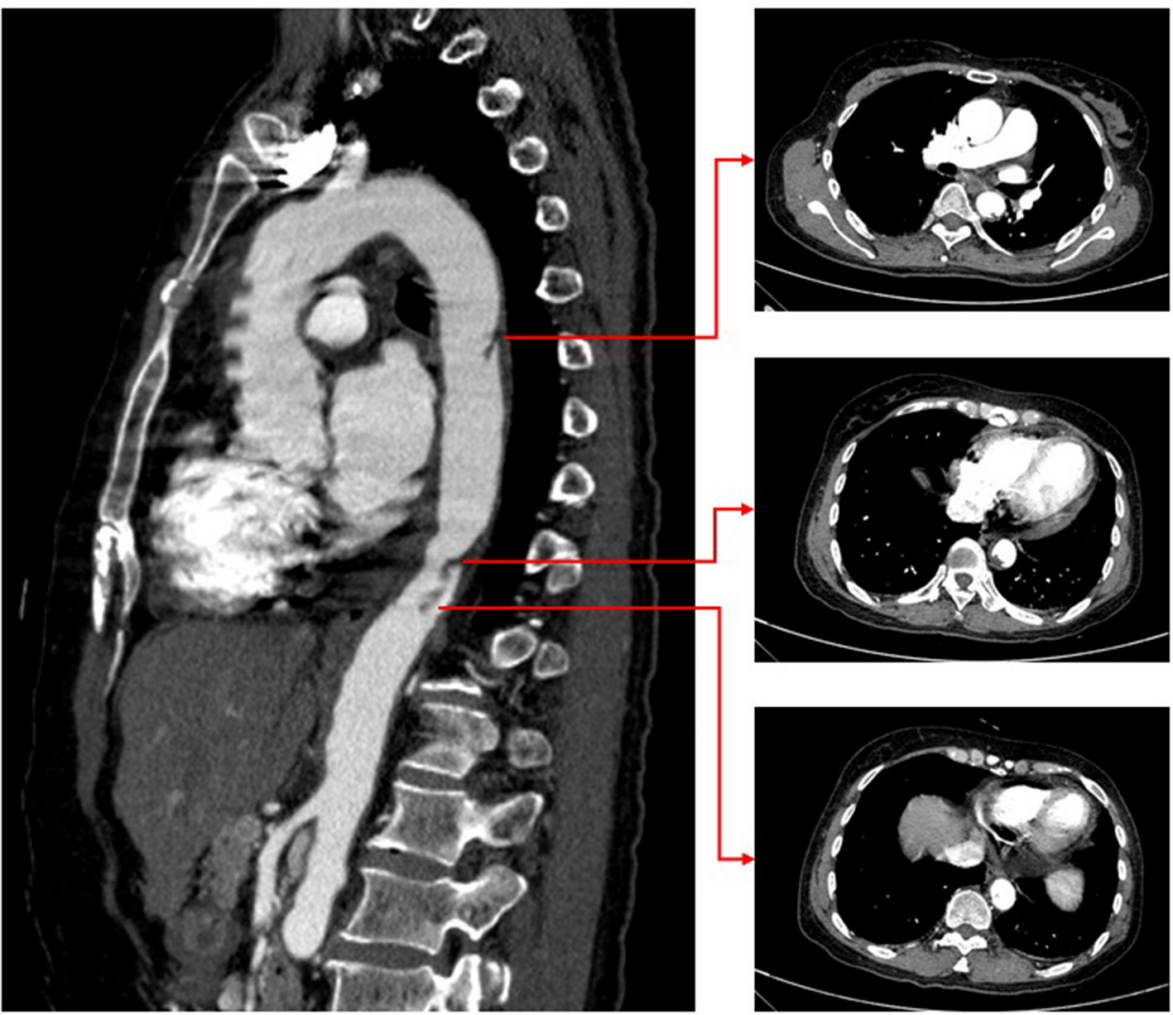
Repeat CT angiography revealing significantly smaller aortic thrombus.
